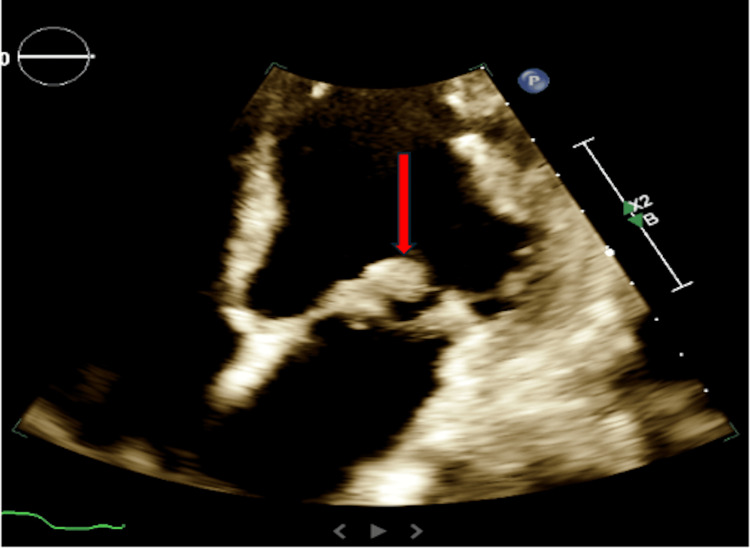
Apical 4-chamber: thickening of the mitral leaflets with a mobile mass attached to the anterior leaflet of the mitral valve
